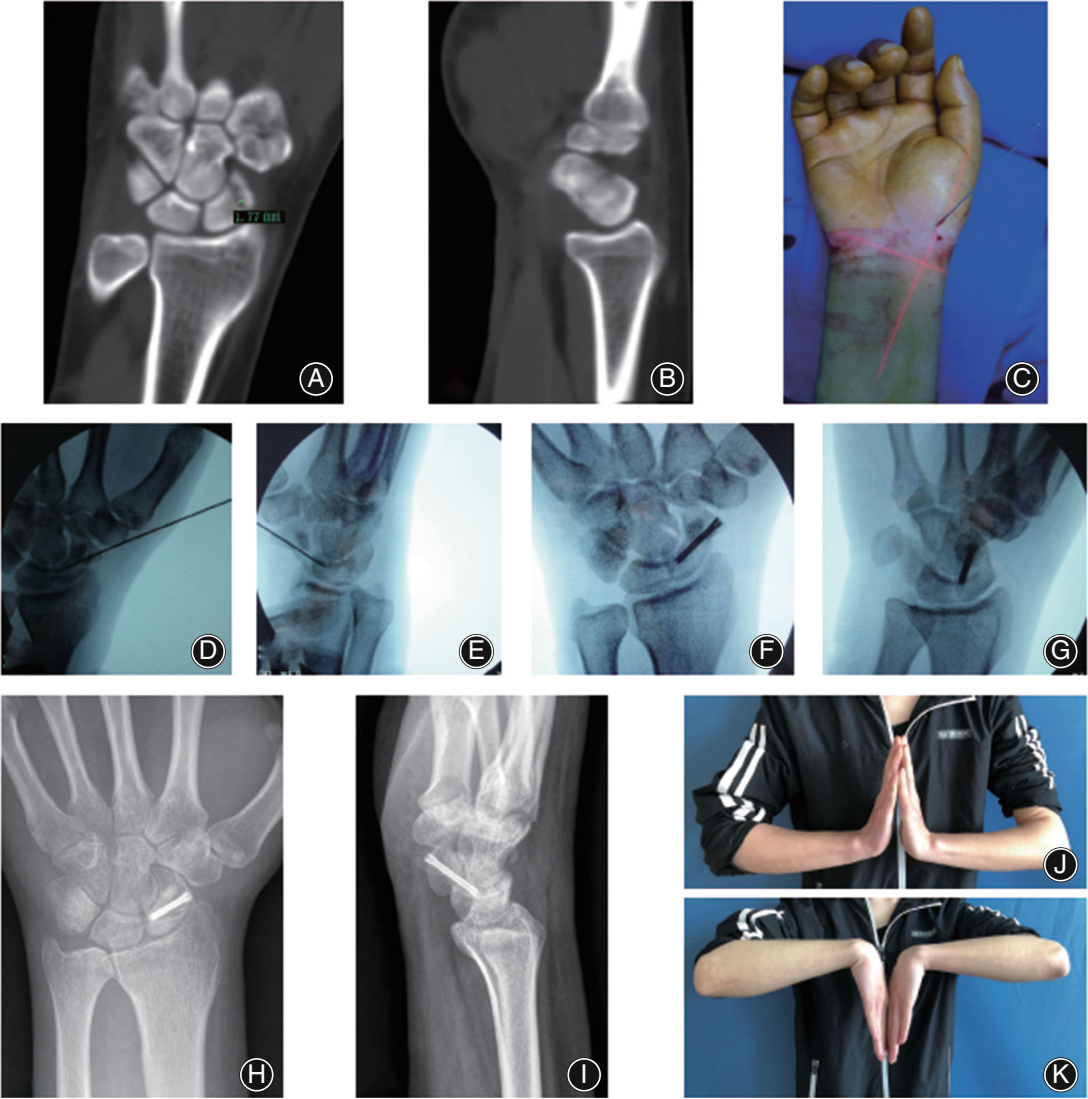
Medical imaging for case 1: A 24‐year‐old male with right wrist pain, swelling, and limited activity for 1 week due to a car accident. (A, B) Preoperative CT showed that the right Herbert B2‐type carpal scaphoid fracture and the separation and displacement of the broken end were 1.77 mm; (C) Closed reduction and temporary guide wire fixation; (D, E) Intraoperative C‐arm fluoroscopy showed that the fracture and guide pin were in good position; (F, G) After screwing in the screw and pulling out the guide pin, C‐arm fluoroscopy showed that the fracture and screw position were good; (H, I) Radiography showed that the fracture line disappeared 12 months after operation; (J, K) 12 months after the operation, the wrist extension and flexion activities were good
